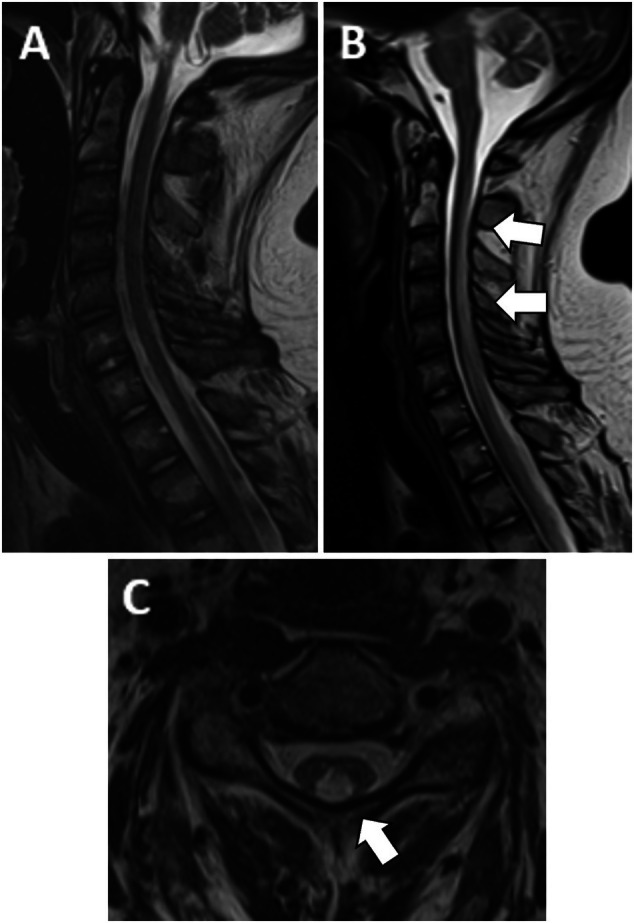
(A) Normal pre‐revumenib sagittal T2WI MRI of the cervical spine. (B) Sagittal T2WI MRI of the cervical spine 4 weeks later (post‐revumenib exposure) demonstrates abnormal signal (white arrows) in the posterior spinal cord. (C) Post‐revumenib axial T2WI MRI images confirm that the abnormality (white arrow) was confined to the posterior columns. This finding extended into the thoracic spinal cord (not shown).
